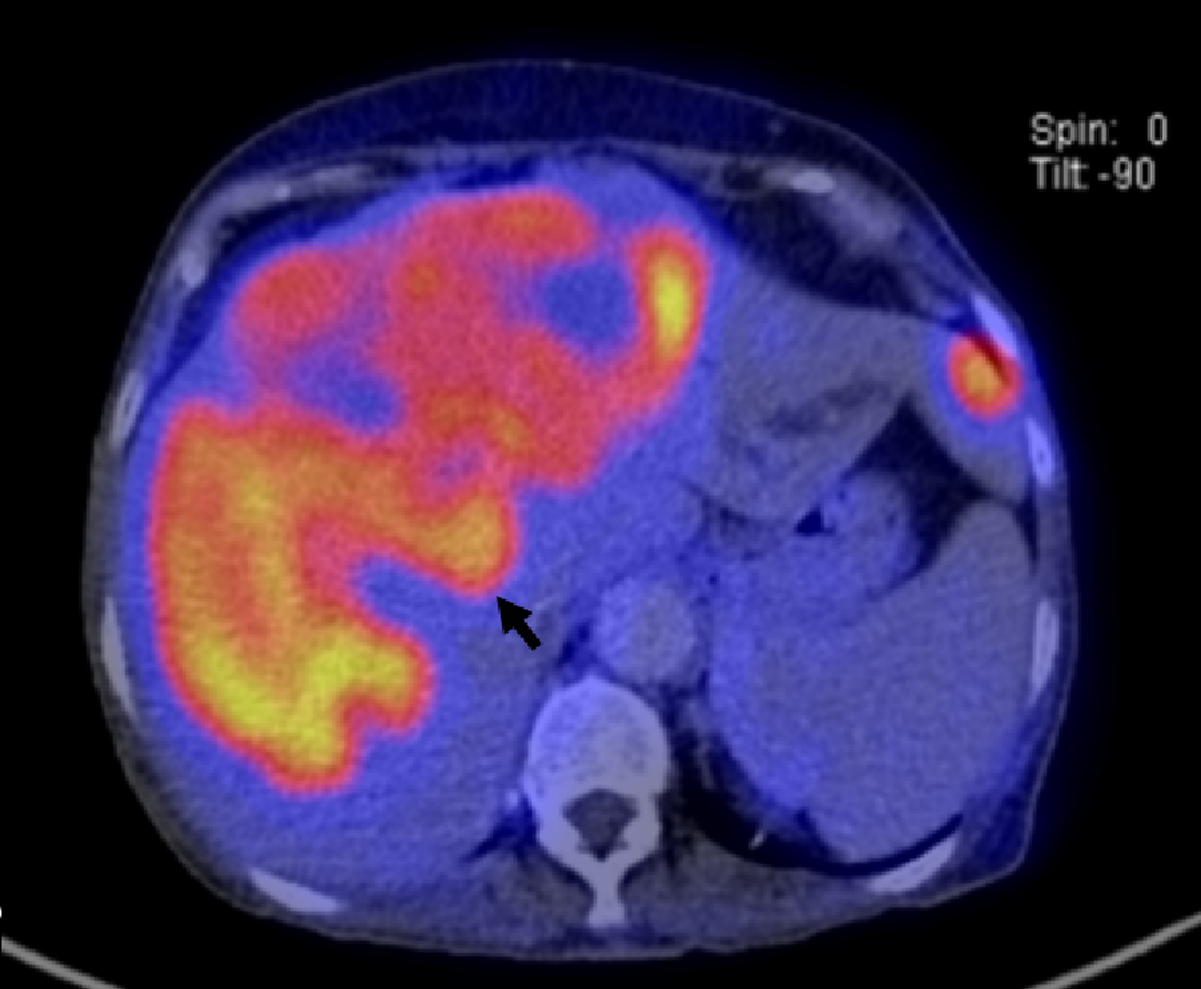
18F-FDG-PET/CT. Hypermetabolic liver lesions, with central convergence and bilateral involvement.
